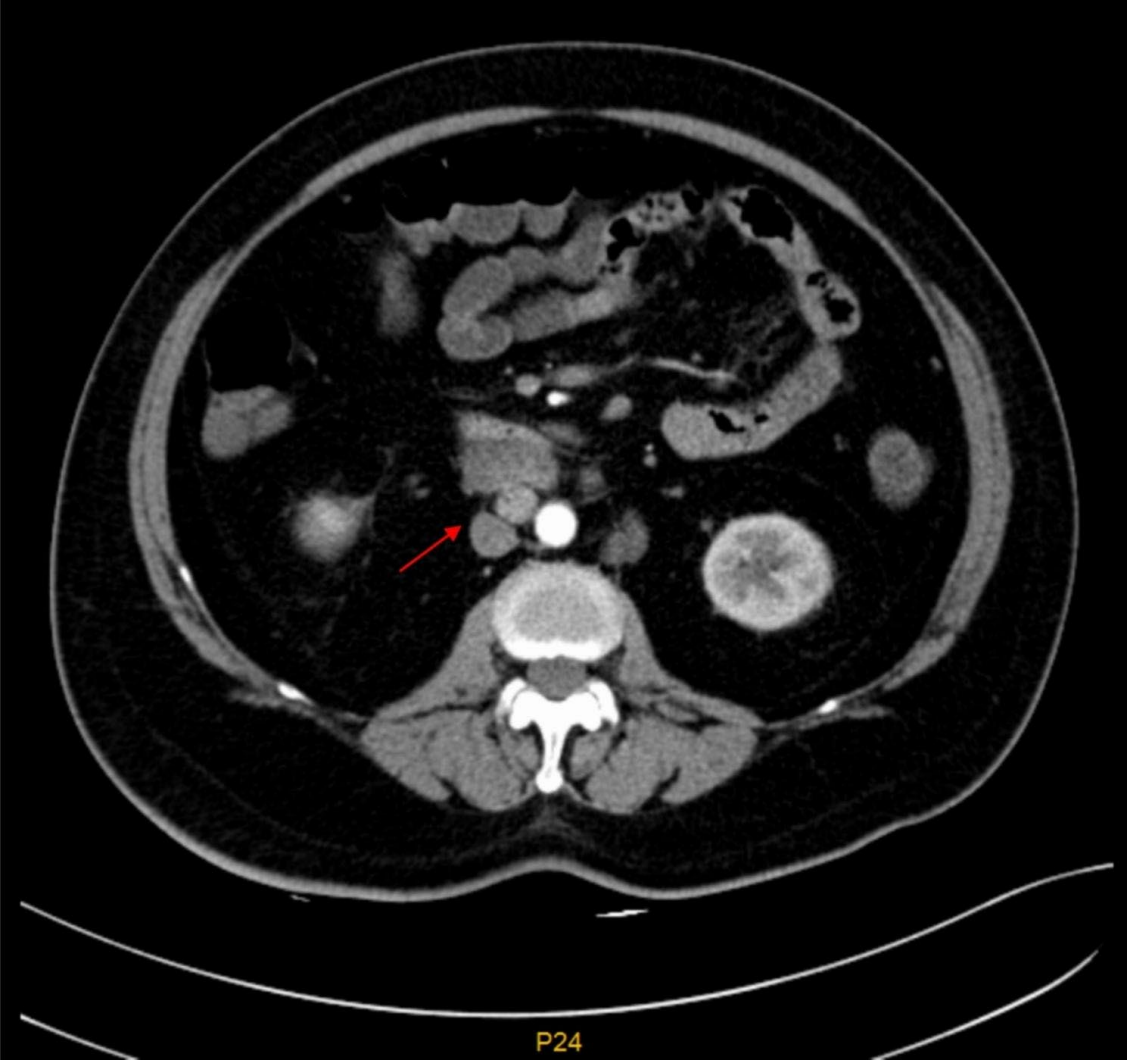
CT abdomen showing multiple retroperitoneal lymphadenopathies. The arrow points to the largest conglomerate of lymphadenopathies (arrow)
